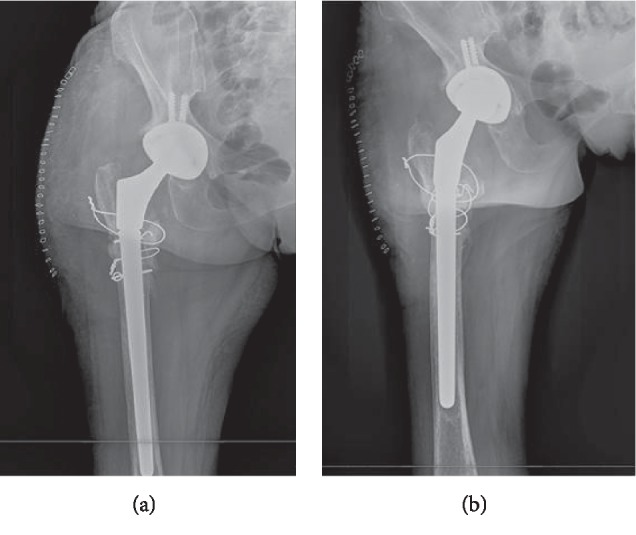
Hip radiography after prosthesis reimplant.
